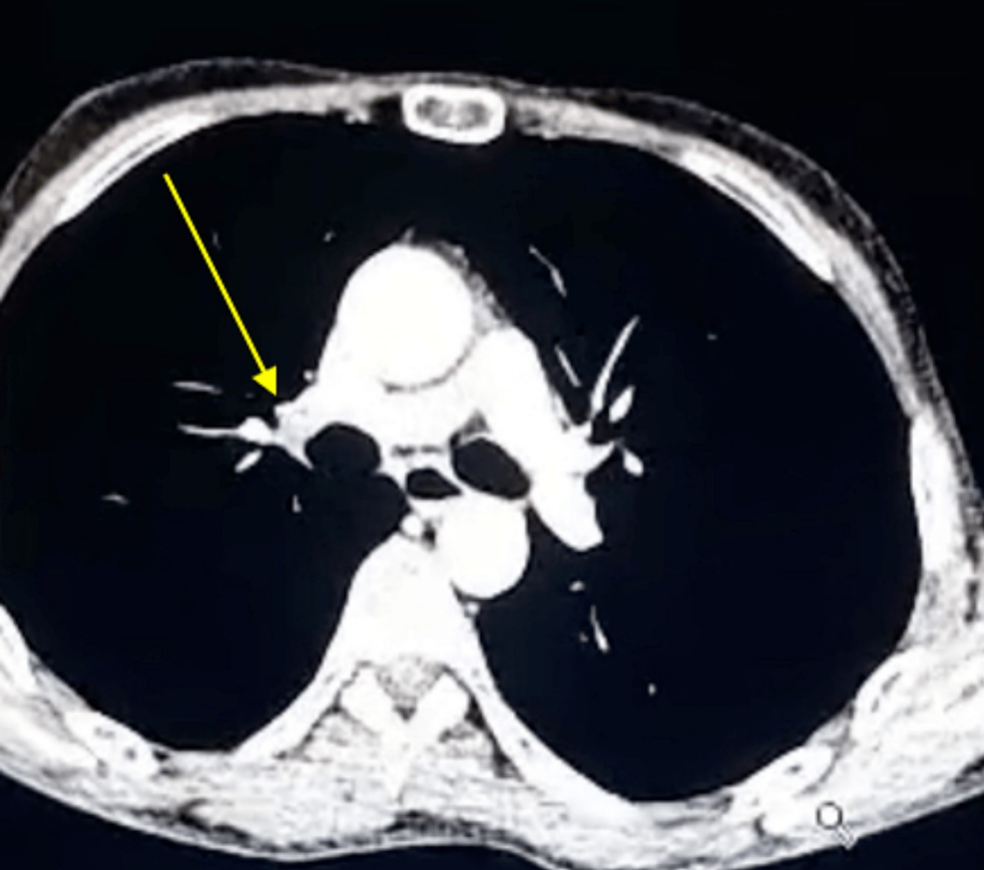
Axial thoracic CT scan (mediastinal window) showing a hypodense material in the right basal segment, consistent with a thrombus. CT: computed tomography
